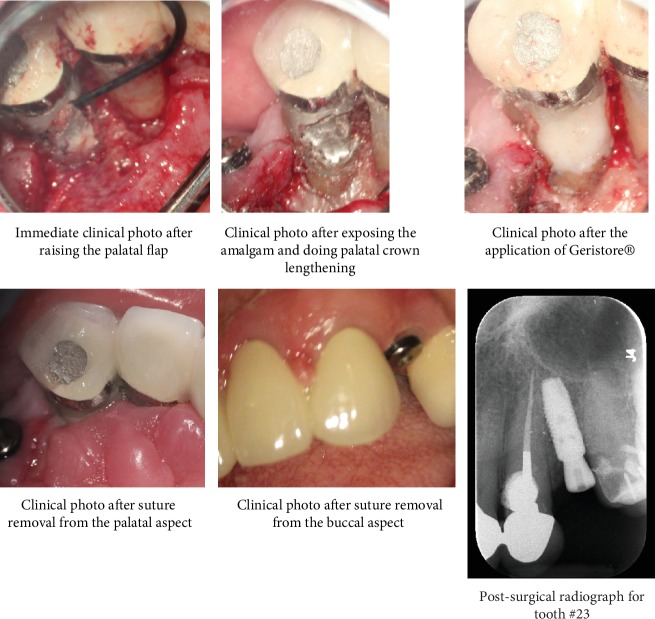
Clinical photos and postsurgical radiograph showing the whole steps for managing the resorptive defect surgically in tooth #23.
